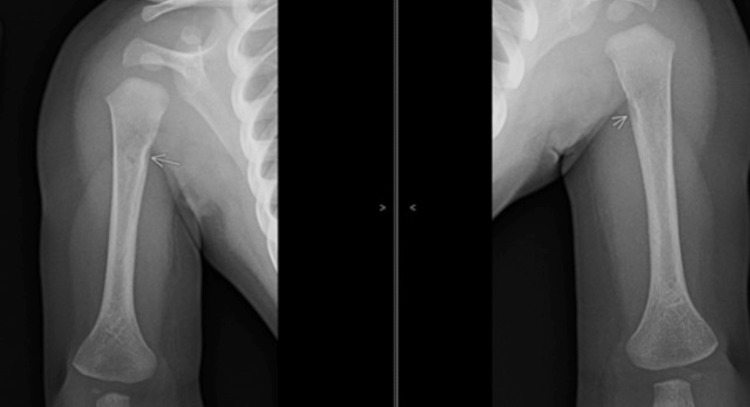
Follow-up frontal radiograph view of the bilateral humeri shows cortical notching involving the medial cortex of proximal bilateral humeral metadiaphysis suggesting a normal variation (arrows).
